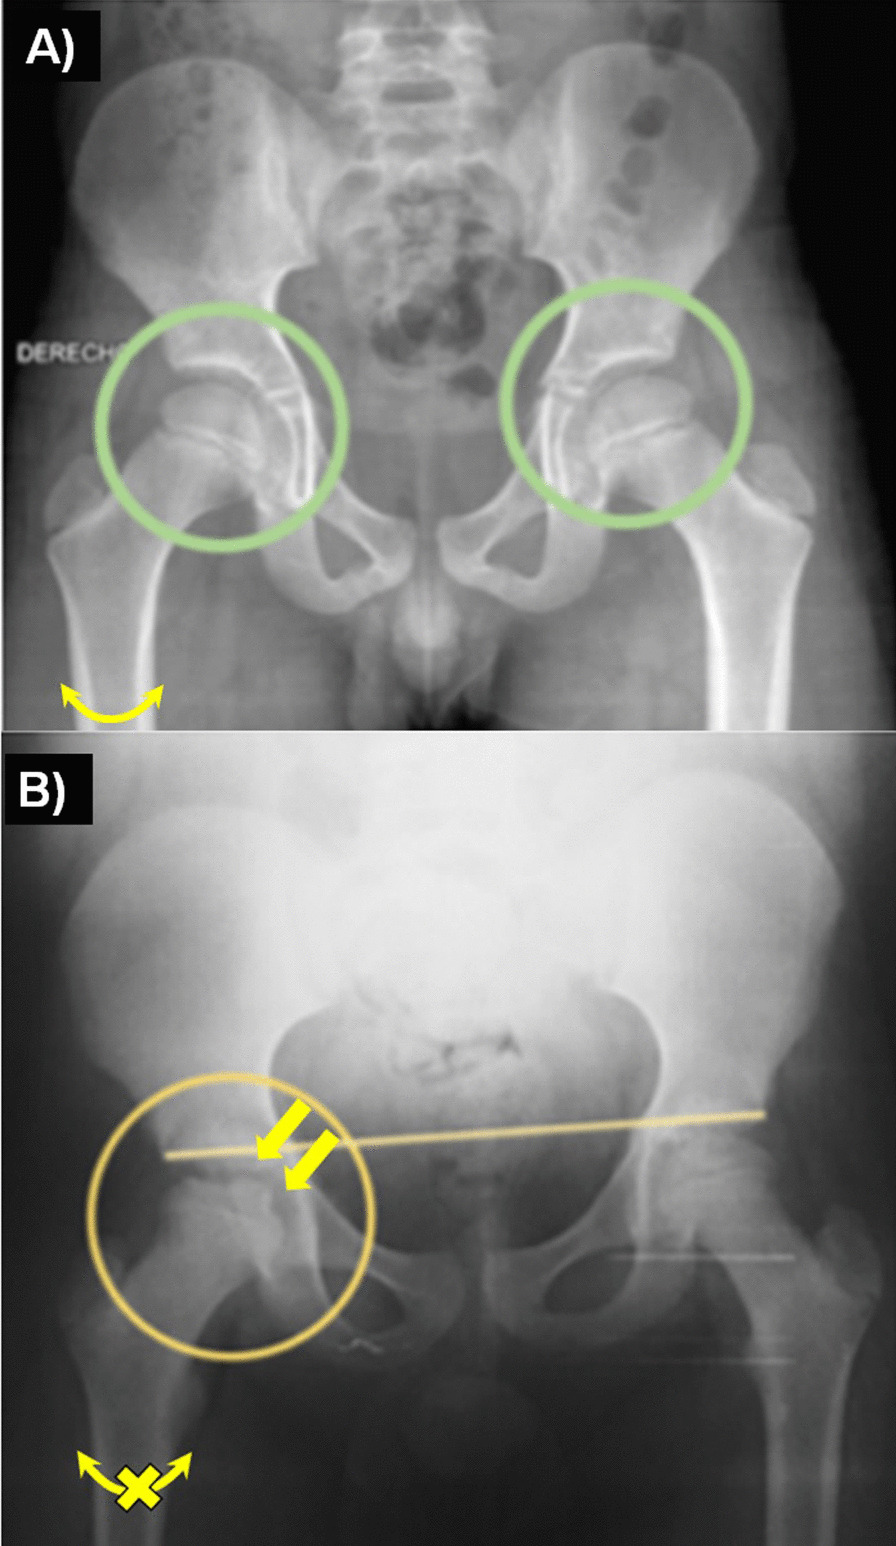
AP radiography. In the AP X-ray the deformity of the hip and femoral head characteristic of LCPD is demonstrable, A healthy control, B LCPD patient. Courtesy of INR-LGII genetics laboratory 2017
